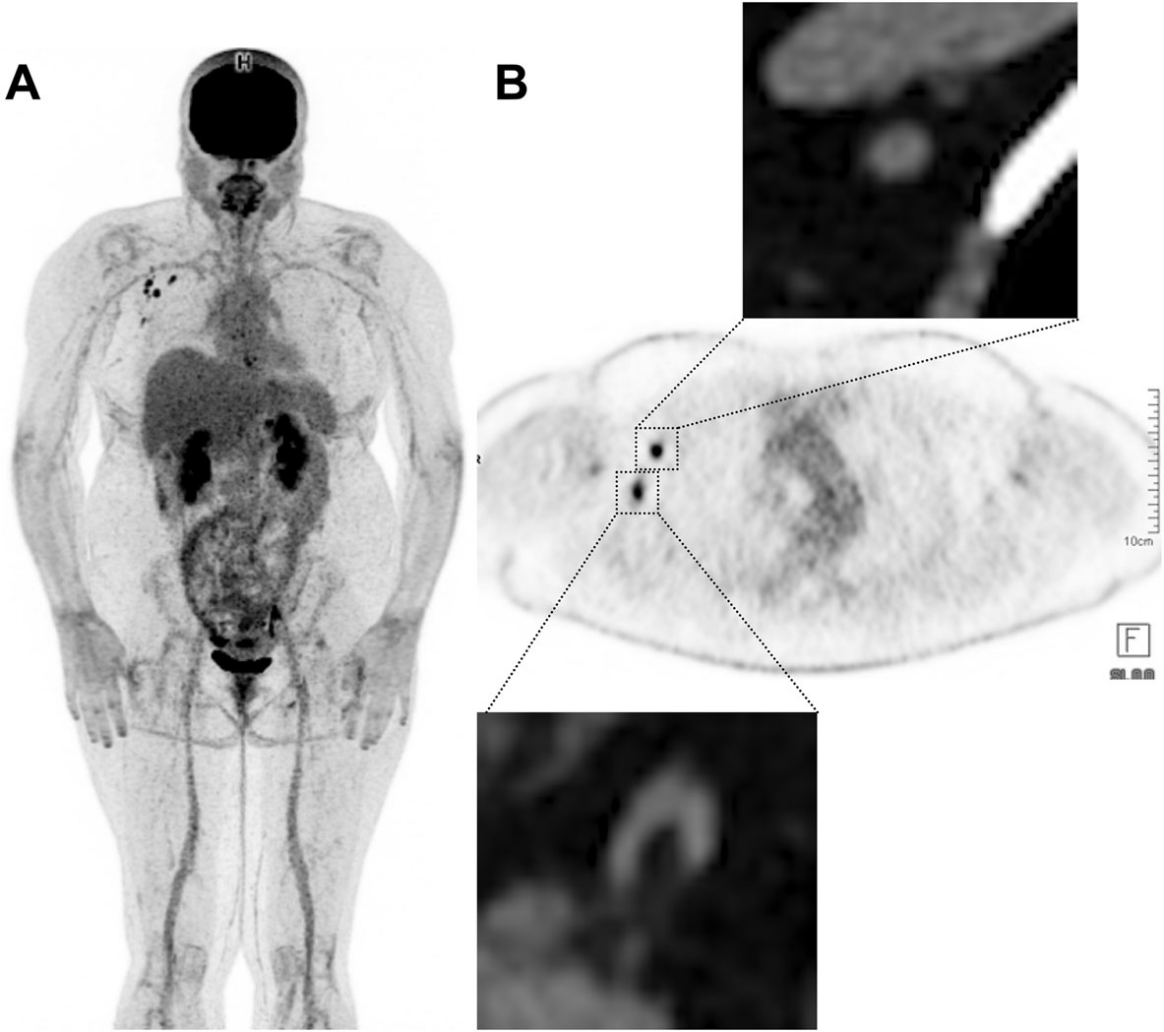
48-year-old woman with previous localization of melanoma on the left knee, who had been treated by TKI for 6 months. This patient received her first dose of COVID 19 vaccine in the right deltoid muscle (Pfizer-BioNtec Cominarty) 21 days prior to the PET examination. Multiple reactive nodes can be seen on the right axillae. Note that some of these nodes have benign CT patterns (bottom right panel: fatty hilum) while others may be considered suspicious based on CT appearance (top right panel: round shape, loss of the fatty hilum pattern). In this case, spread to the contralateral axilla from a leg primary without regional nodal or distant metastatic involvement should alert the reader to a low likelihood of malignancy
